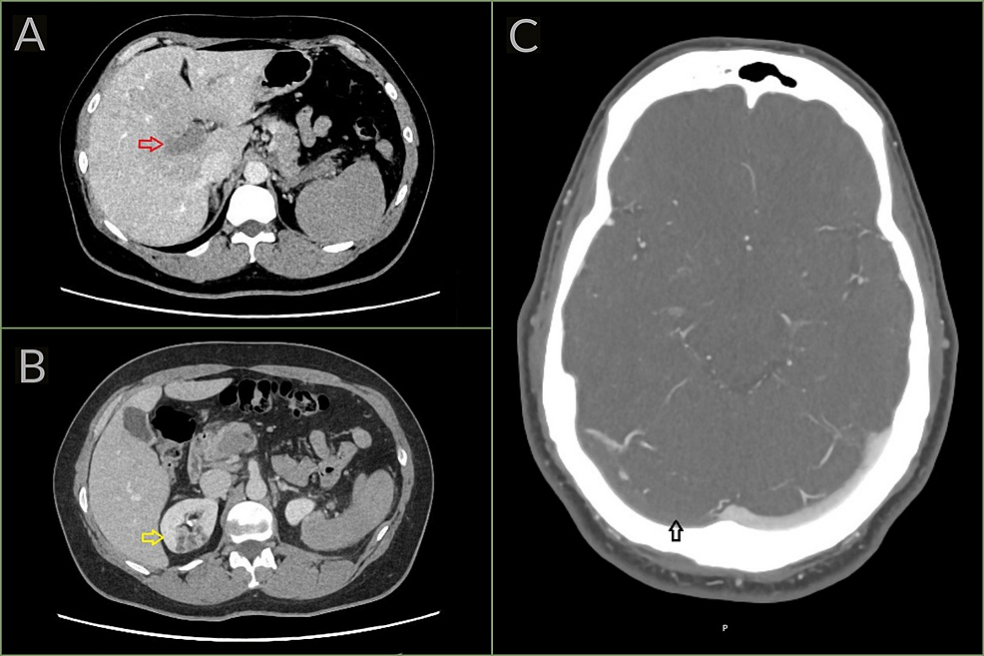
(A and B) CT of the abdomen with contrast and (C) CT of the head with venogram (Case 2). (A) There is complete occlusion of the portal vein and its branches (red arrow). (B) A wedge infarct is shown in the upper pole of the right kidney (yellow arrow). (C) The CT head with venogram (CTV) demonstrates occlusive thrombus within the right transverse sinus (black arrow). In comparison, the left transverse sinus is patent and completely opacified.
